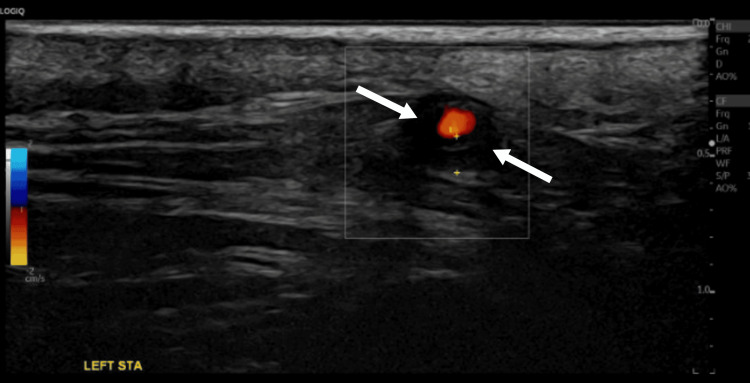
The left STA US Doppler Left STA showing circumferential hypoechoic wall thickening and periluminal dark "halo sign" (arrows) STA: superficial temporal artery; US: ultrasound
